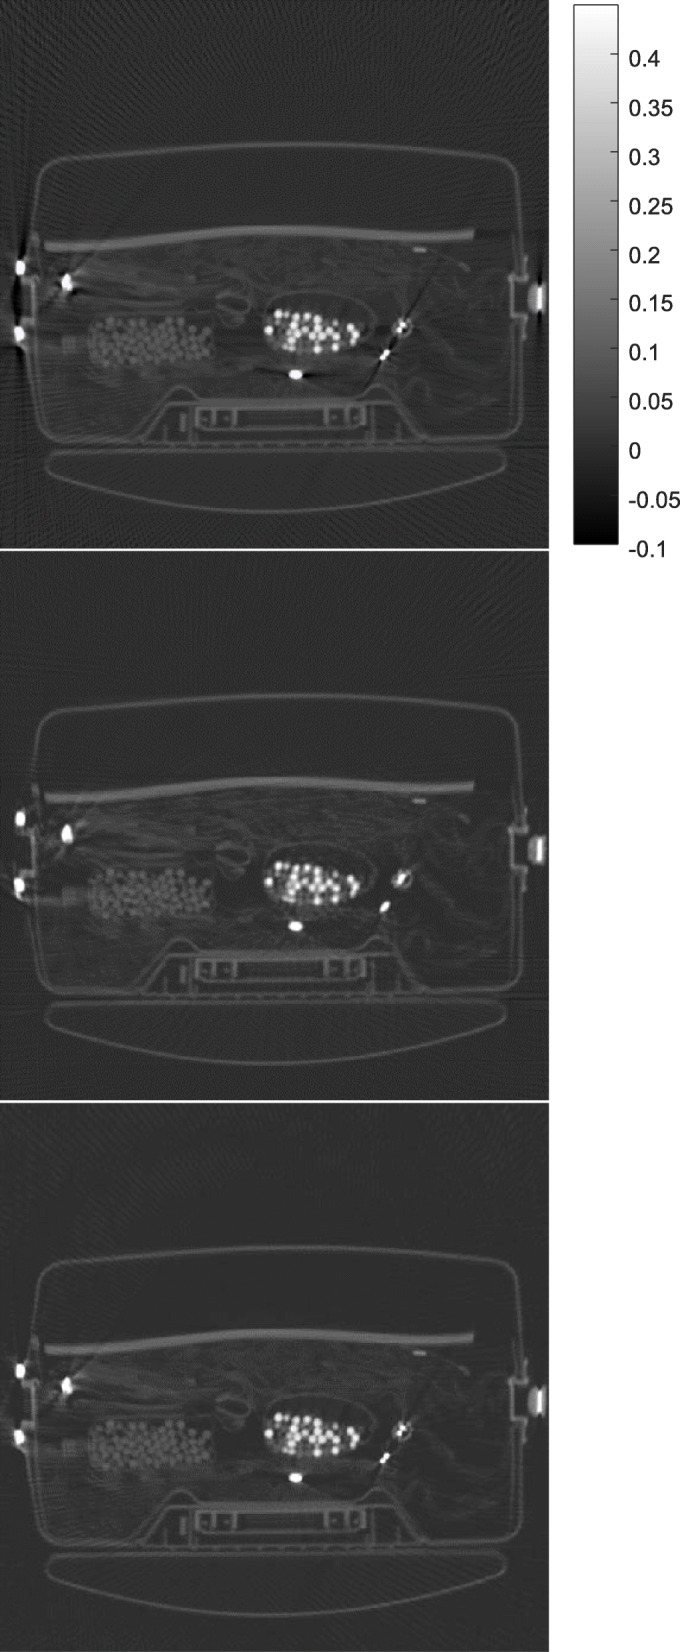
CT image reconstruction of airport bag #4. Top: the raw FBP reconstruction; Middle: the reconstruction using the proposed algorithm; Bottom: the raw FBP reconstruction with all negative pixel values replaced by zeros
